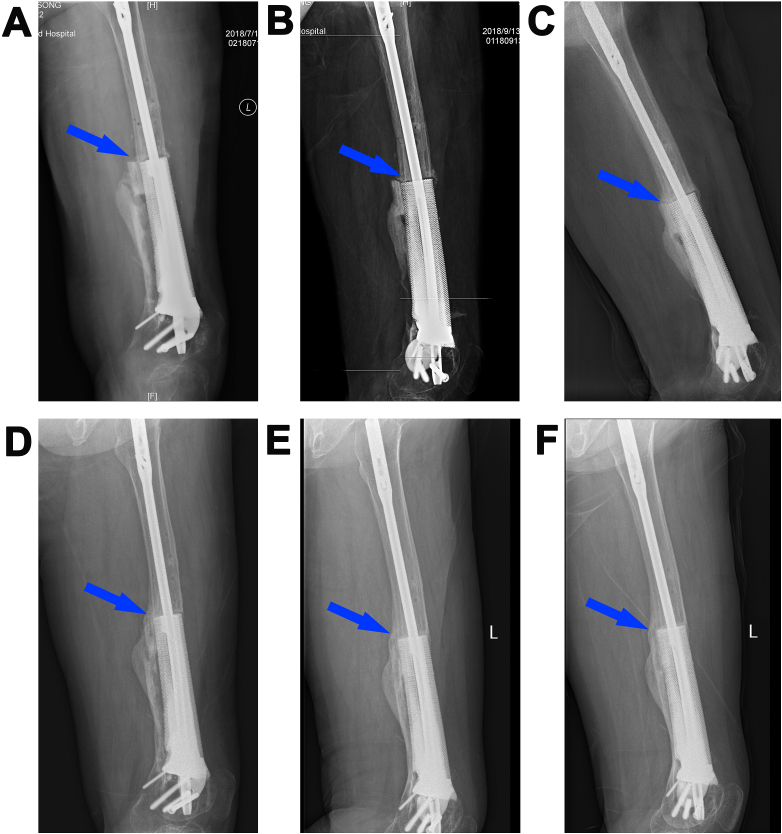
A femur defect reconstructed by a three-dimensional (3D)-printed porous Ti6Al4V implant (Case 2). Radiographs of the reconstructed 11-cm femur defect after the last surgery (A), and at 2 (B), 5 (C), 8 (D), 14 (E), and 20 (F) months after implantation. Blue arrows indicate the osseointegration between the scaffold and host bone.
